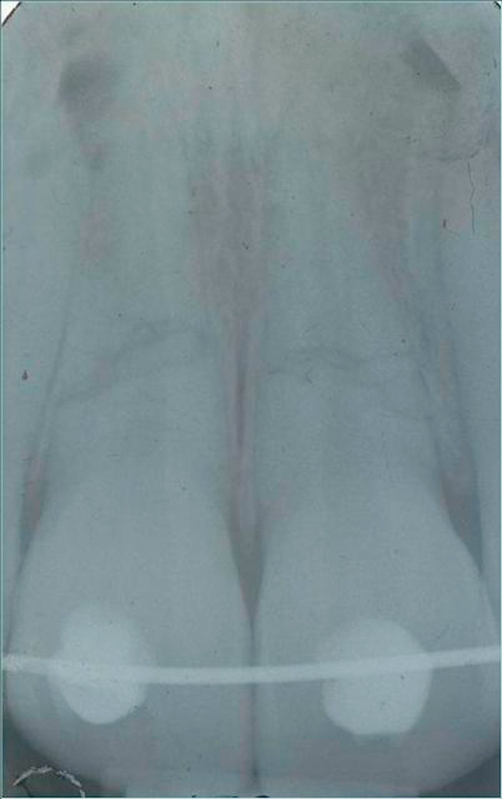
Radiographic control at 3 months.
